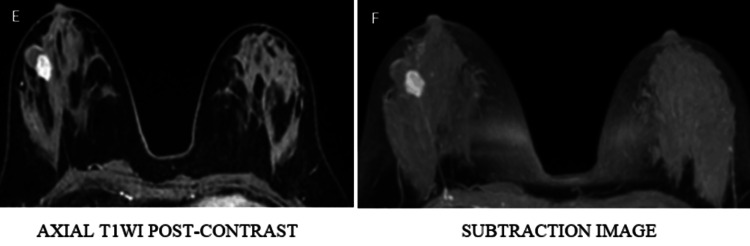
Dynamic post-contrast imaging (E, F) demonstrates intense enhancement of the solid component T1WI: T1-weighted image
